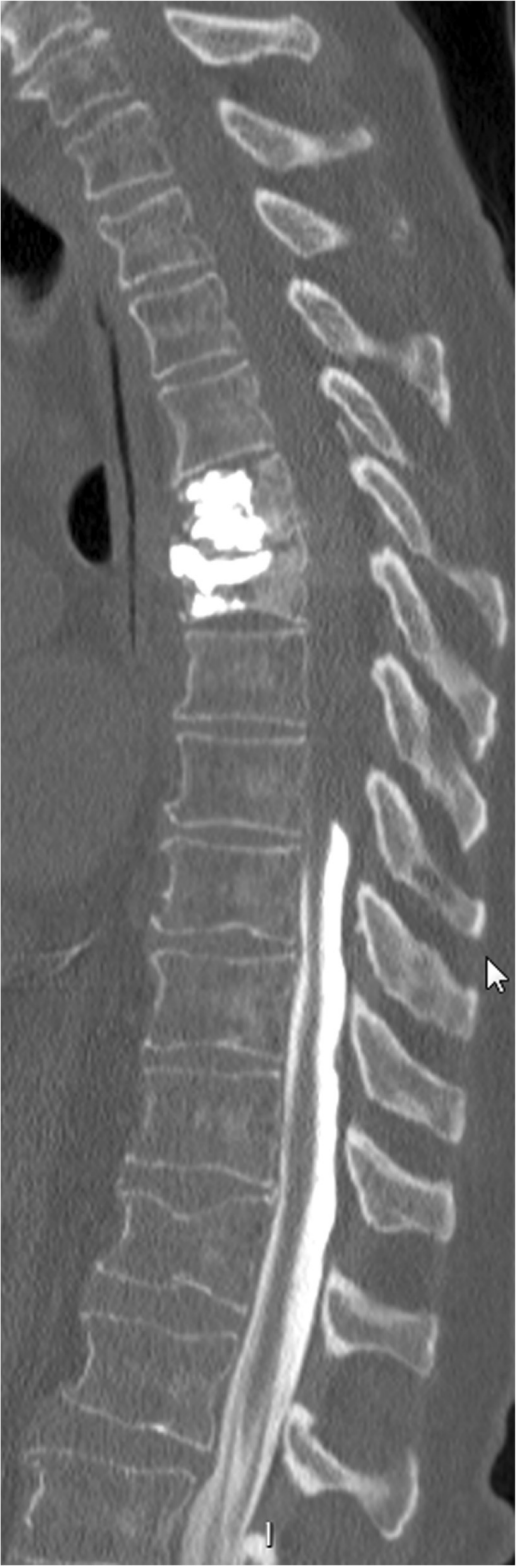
Spine CT shows a block from the level of eight thoracic vertebrae due to an epidural posterior mass
